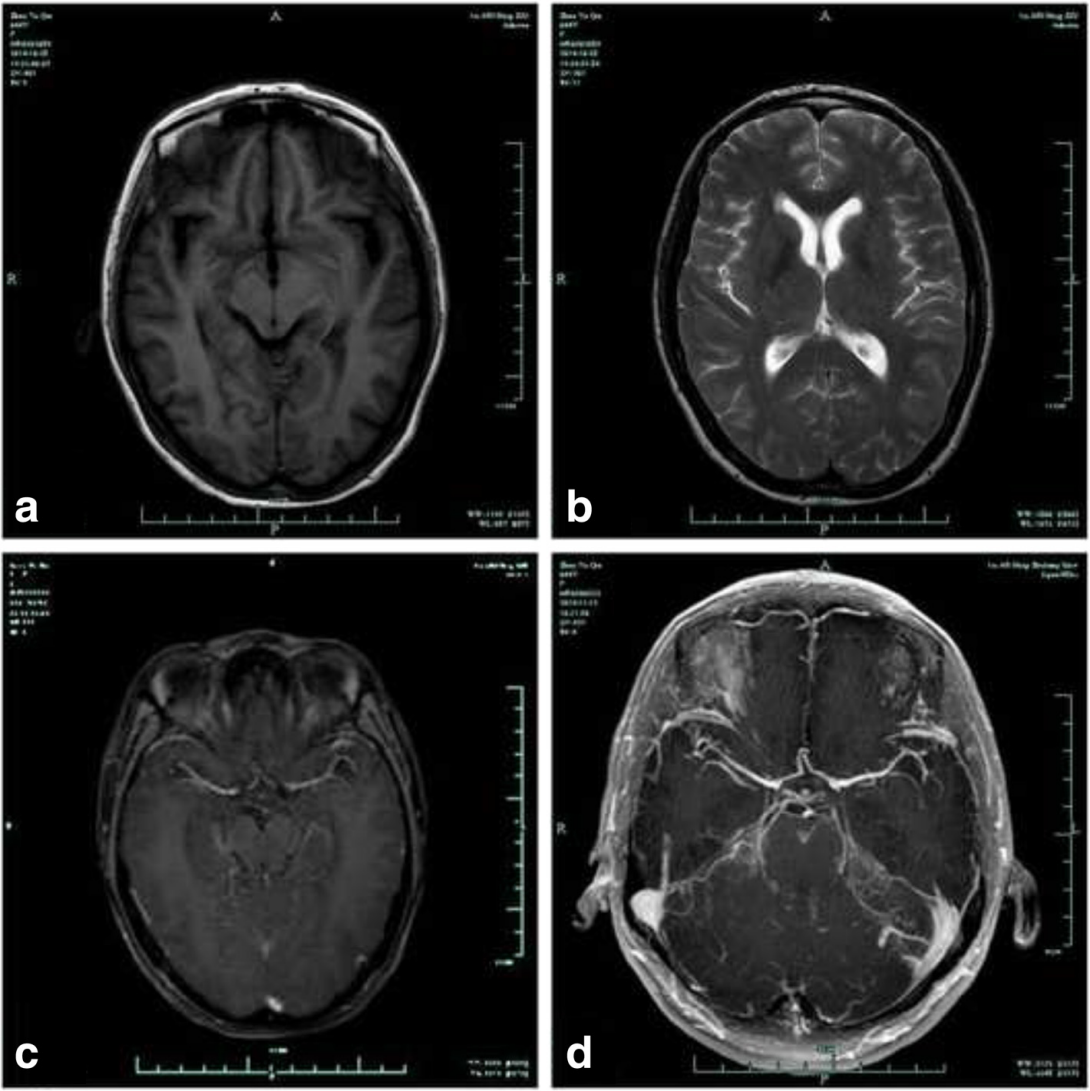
Axial magnetic resonance imaging of brain: T1-weighted imaging (a), T2-weighted imaging (b) and Gadopentetate dimeglumine-enhanced T1-weighted image (c) do not detect any solid tumor or enhancement meninges. While the gadobutrol-enhanced T1-weighted image shows serpentine enhancement along the surface of the brain (d)
